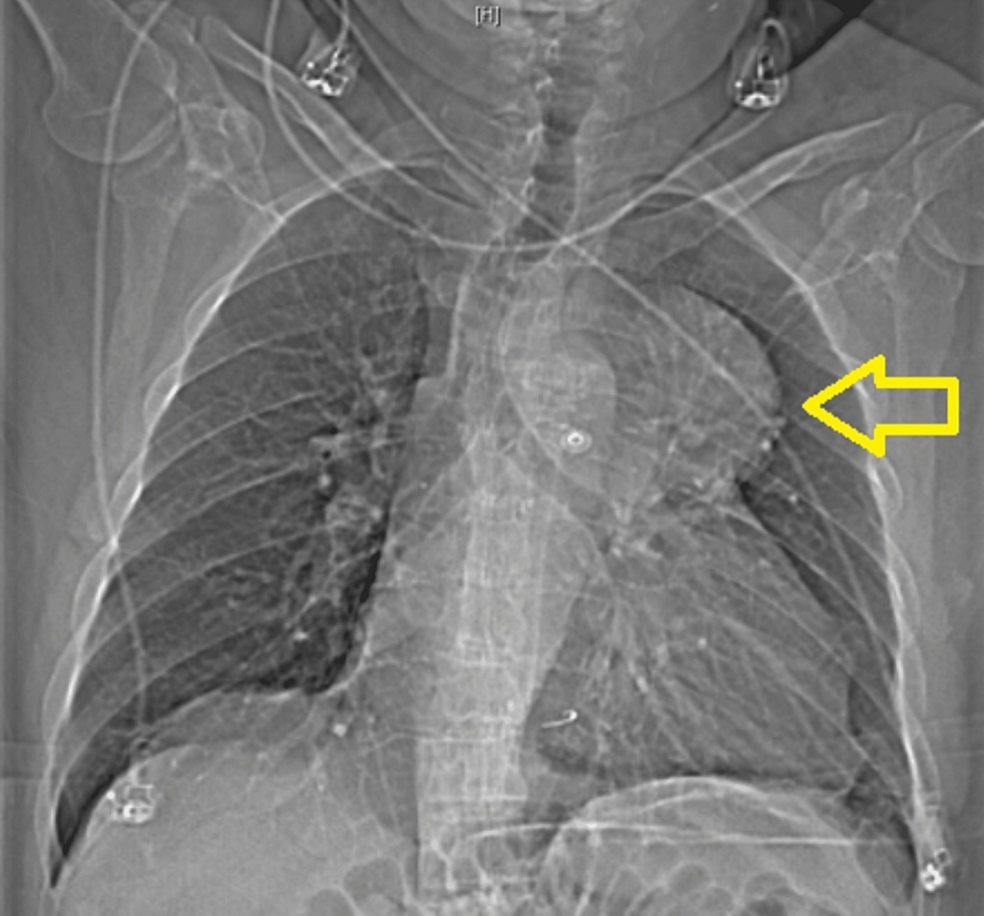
Chest X-ray Demonstrating Suprahilar Mass The arrow indicates the suprahilar mass.
